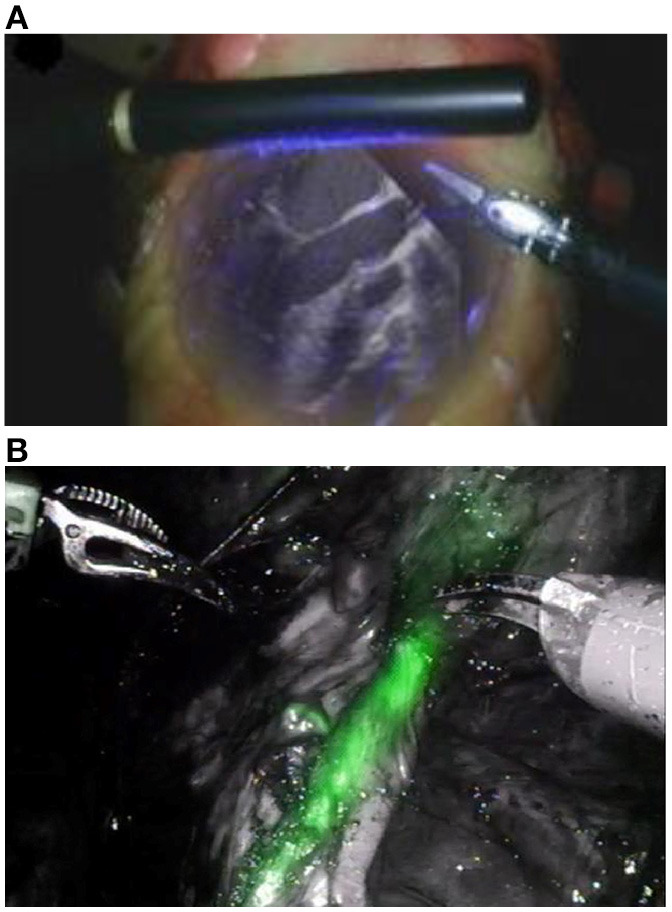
(A) Augmented visible light and ultrasound image from Intuitive Surgical's Da Vinci ultrasound probe (Lee et al., 2010) and (B) visible light overlaid with NIRF from the Pinpoint Novadaq system (Kaplan-Marans et al., 2019). Both images are taken from real time video. Reproduced with permission from Elsevier.
